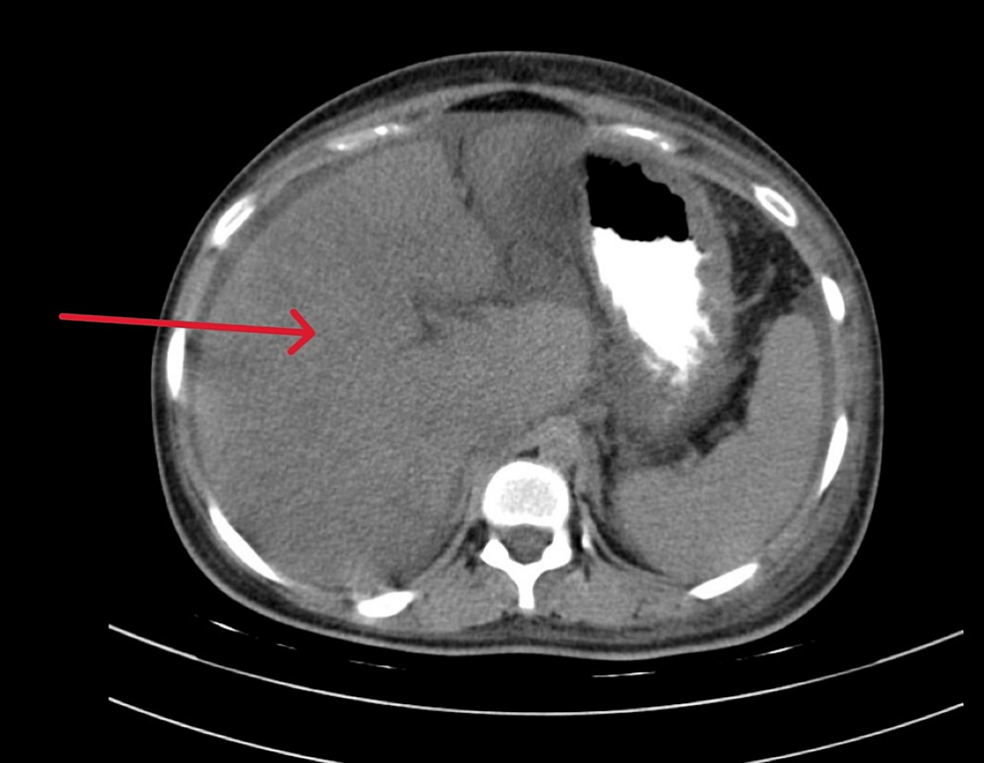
CECT of the abdomen and pelvis showing altered echotexture of the liver (indicated by the red arrow). CECT: Contrast-enhanced computed tomography.
